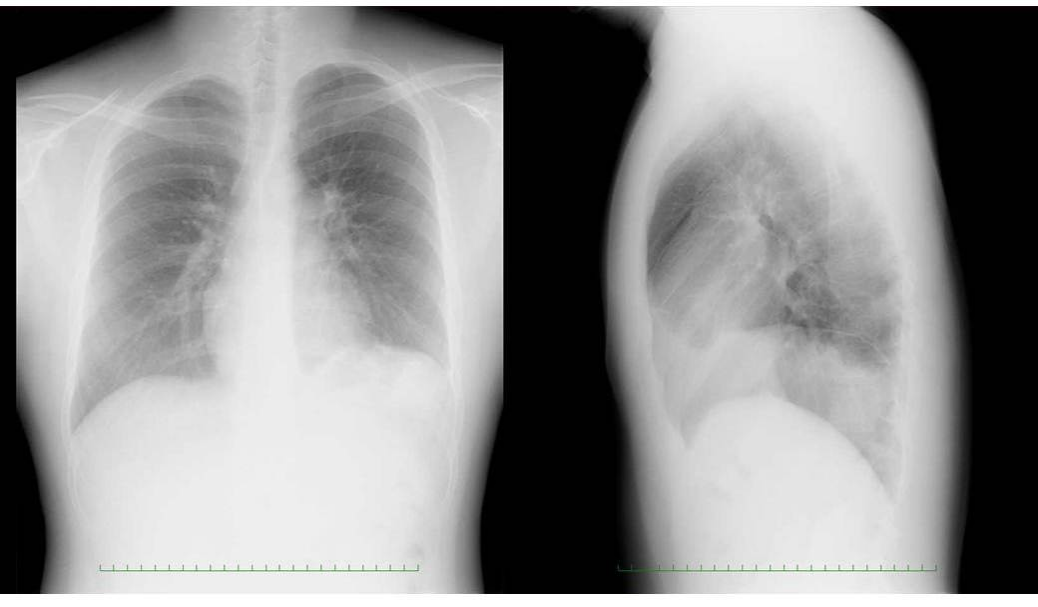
Preoperative chest radiograph. The chest radiograph shows elevation of the left diaphragm. In this case, the lateral chest radiography was important for the detection of an abnormality in the thoracic cavity.
